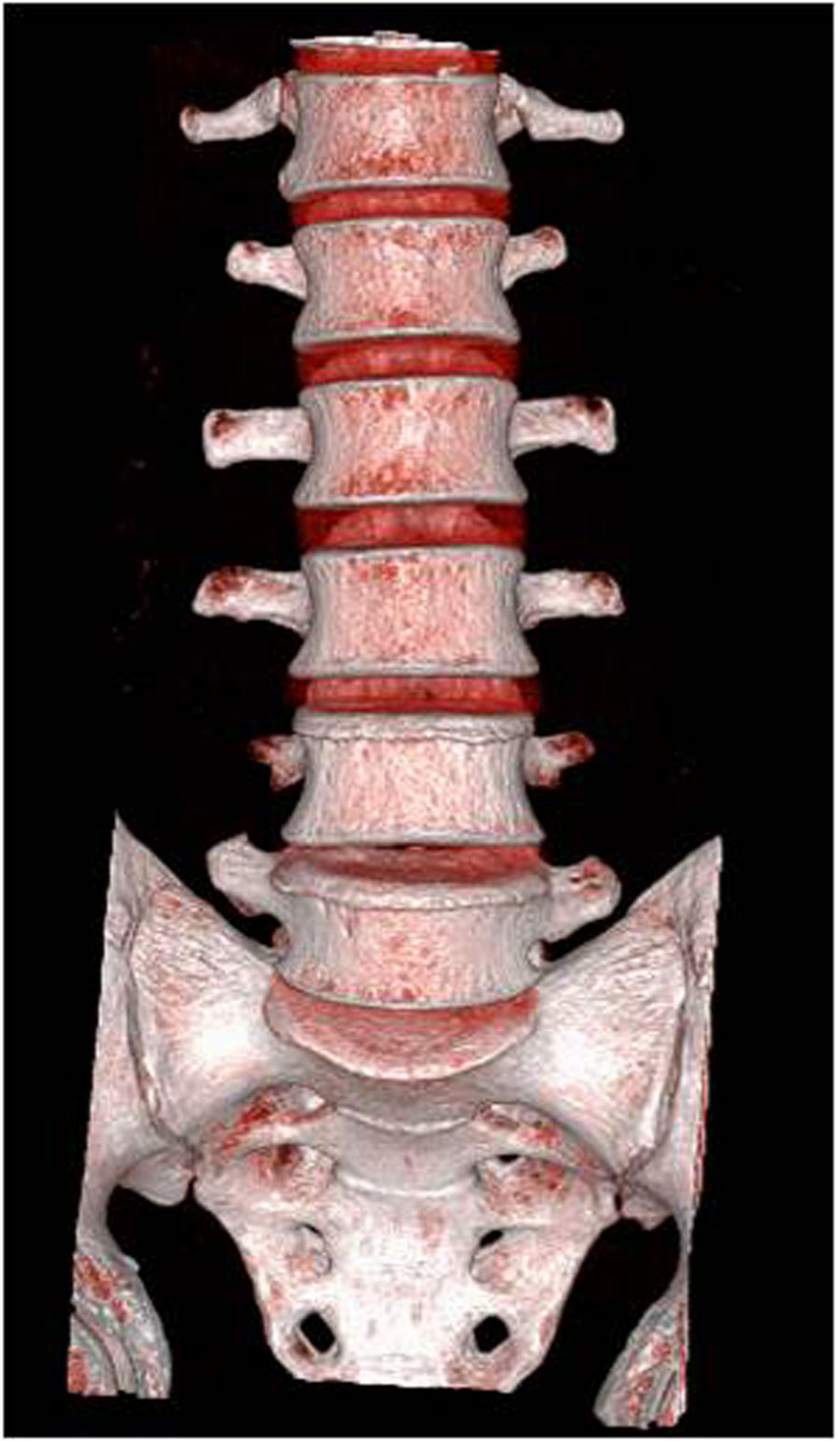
A CT scan was taken from the lower endplate of 12th thoracic vertebra (T12) and below the 1st sacral vertebra (S1) with a 1-mm thickness slice
